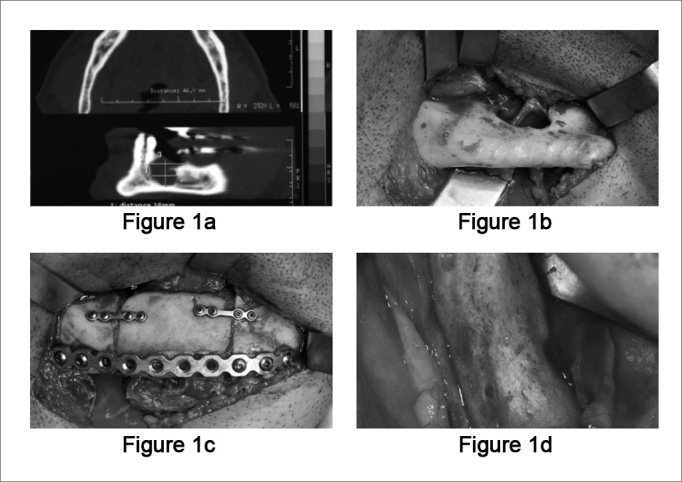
Mandible CT scan, showing the ameloblastoma destroying part of the mandibular cortical bone. Figure 1b. Intraoperative photograph, with submandibular access showing mandibular cortical bone erosion caused by the ameloblastoma, notice the exposed mandibular nerve. Figure 1c. Intraoperative photograph, showing osteosynthesis with titanium plates and screws. Figure 1d. Intraoperative photography (8 months after the graft), showing complete graft integration.
